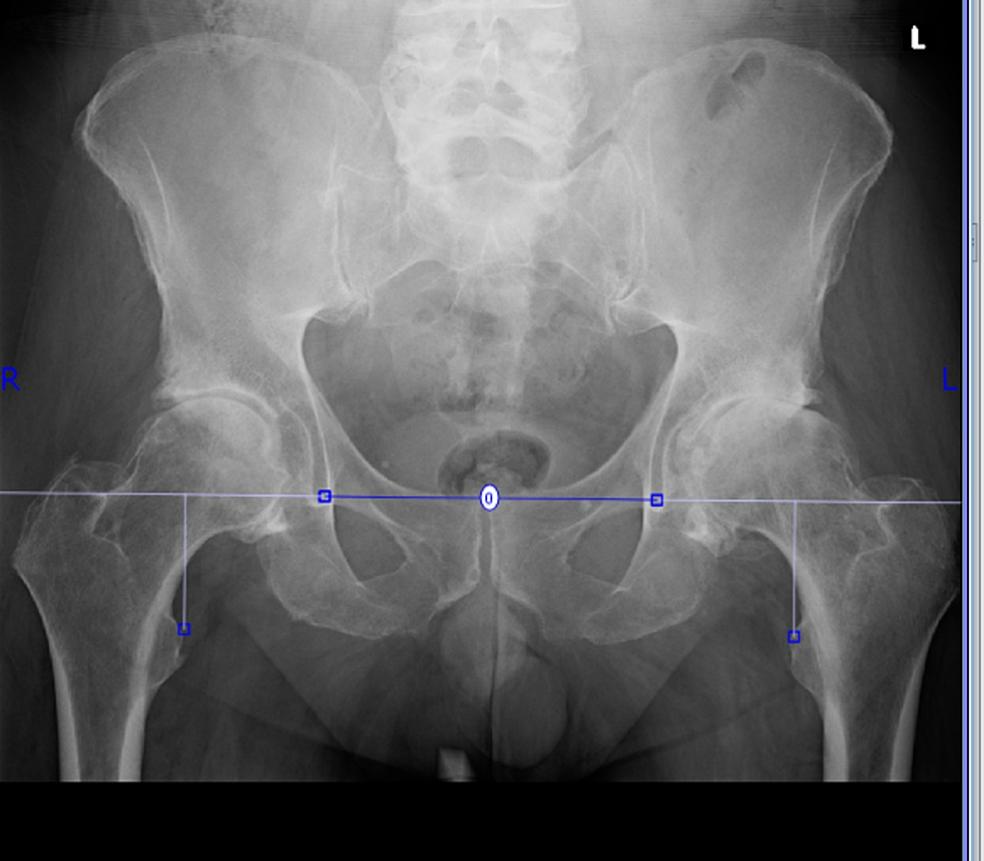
A preoperative anteroposterior pelvis radiograph of THA The image shows the limb length measurement as the vertical distance between the inter-acetabular teardrop line and the most prominent medial point of the lesser trochanter using the OrthoView Workstation^TM^ THA: total hip arthroplasty
